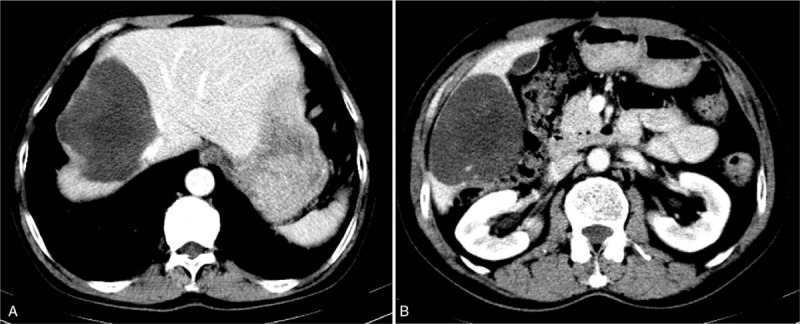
Tumor size on computed tomography (1 year after operation): the multiple liver lesions were smaller than before. (A) 8.4 × 10.3 cm. (B) 9.4 × 7.3 cm.
